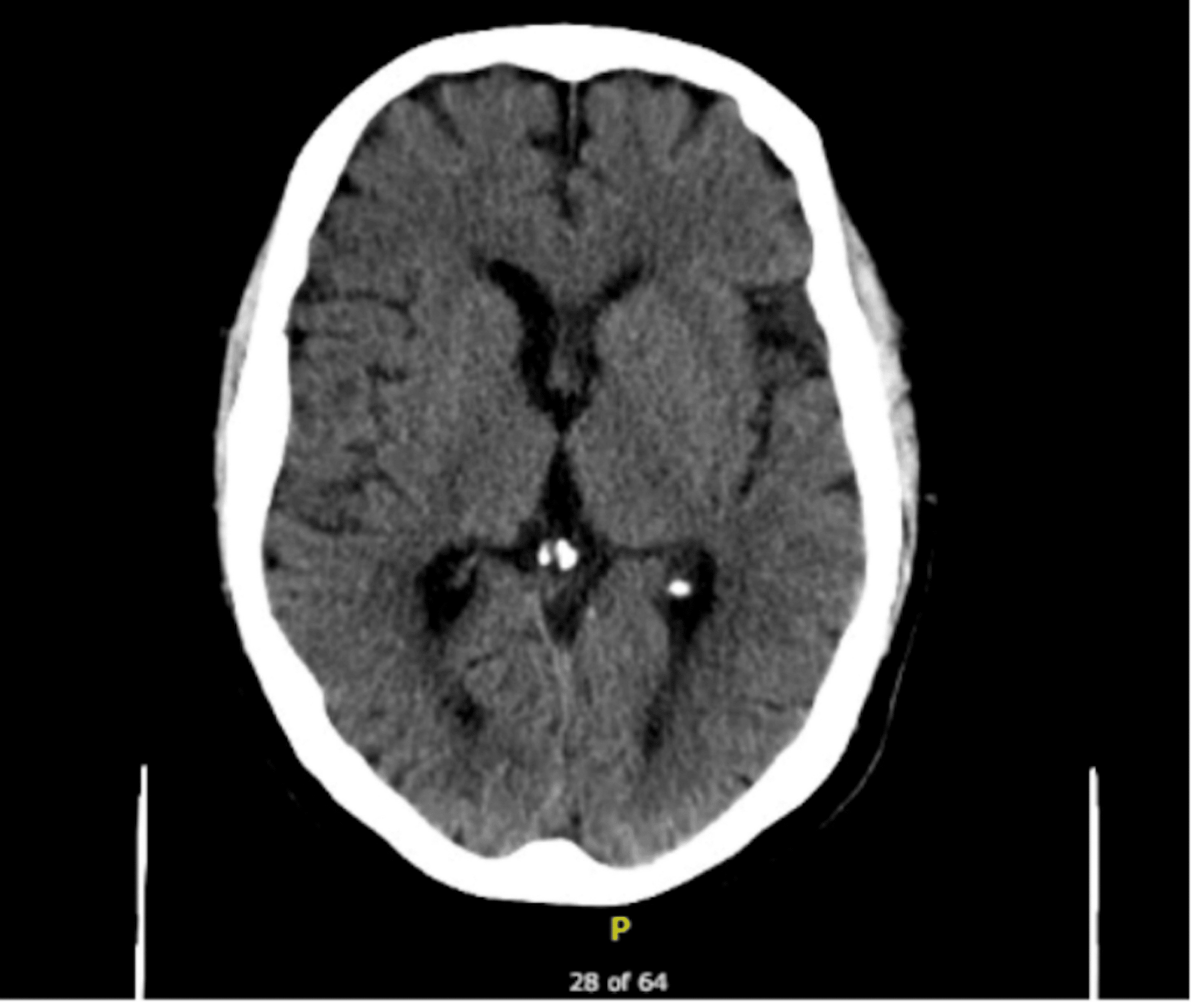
CT head showing no acute intracranial pathology that would explain seizure activities.
